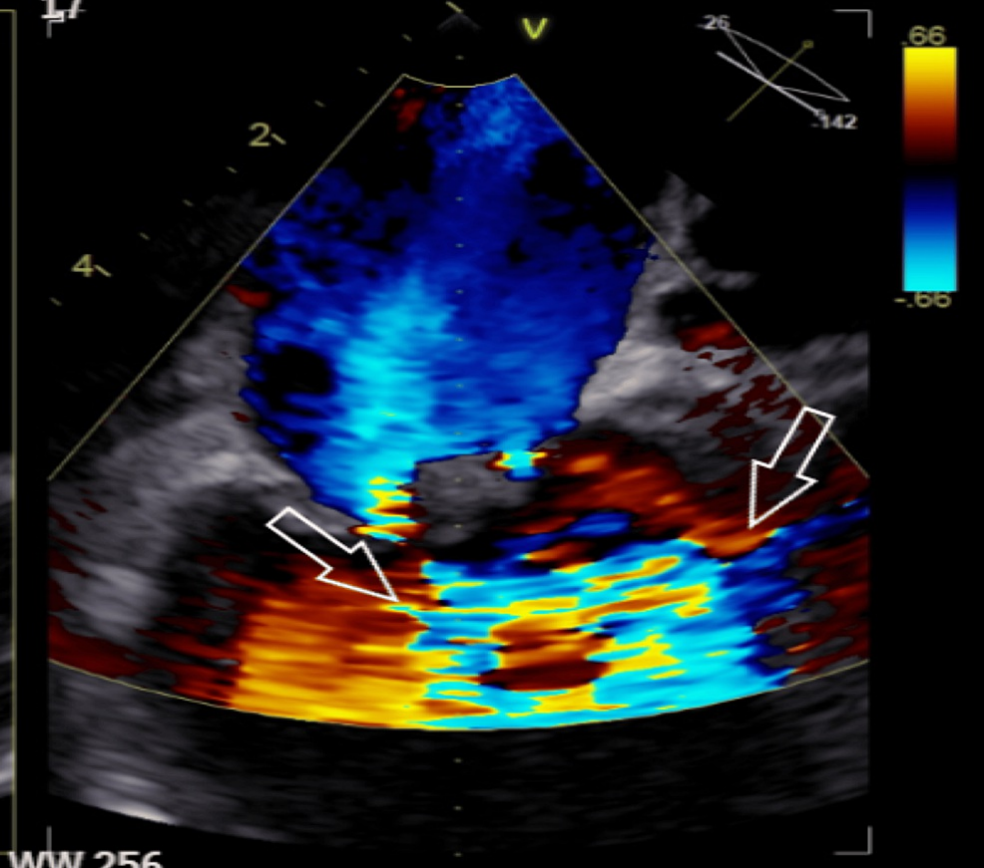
Transesophageal echocardiography shows moderate mitral regurgitation
